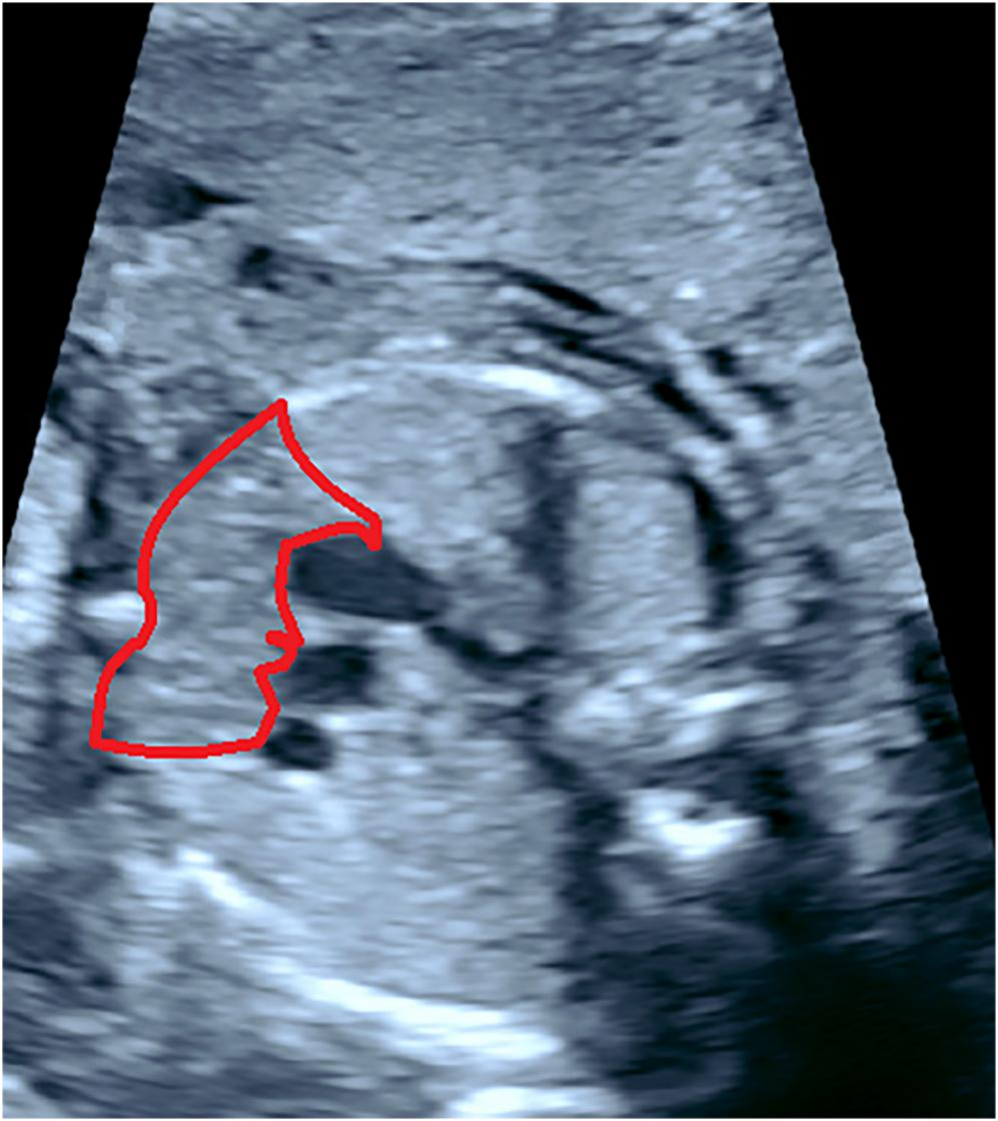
Ultrasound image of the fetal thymus at the level of the three vessel view
